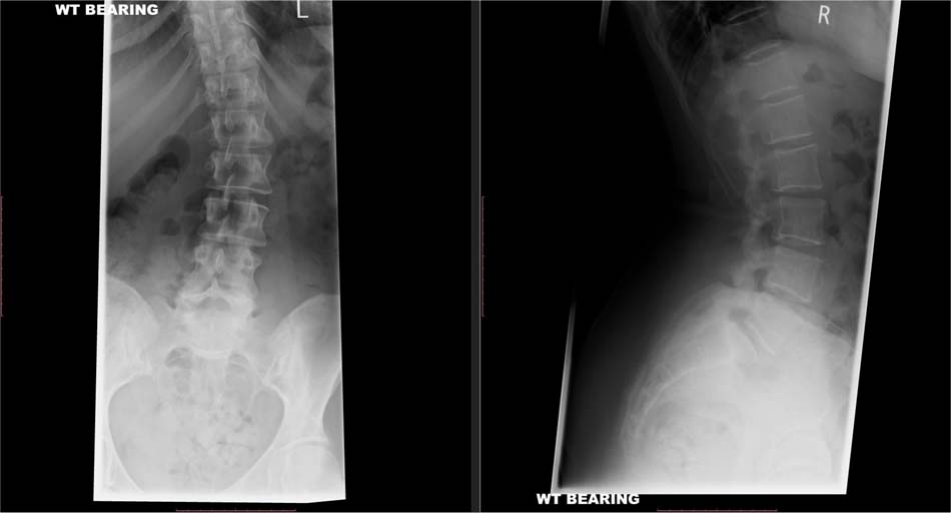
Preoperative X-ray of the lumbar spine performed in December 2015, confirming small idiopathic scoliosis in the lumbar spine.
